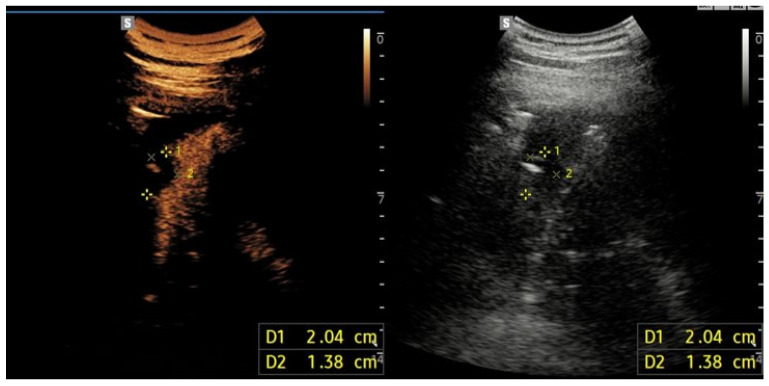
Contrast-enhanced ultrasound features of lobar pneumonia with intraparenchymal necrosis and associated pleural effusion. Contrast-enhanced ultrasound (left) and corresponding B-mode ultrasound (right) images show a lobar pulmonary consolidation with a well-defined, non-enhancing intralesional area consistent with necrosis (measured diameters indicated). A concomitant ipsilateral pleural effusion with complex, echogenic content is also observed.
